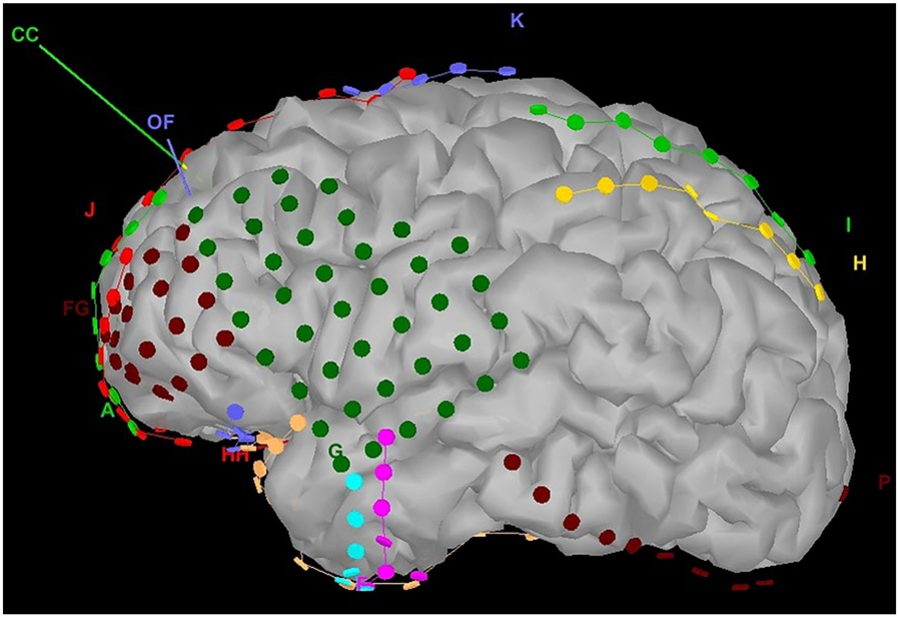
Reconstruction of electrodes on a mesh of a preimplantation 3-D reconstruction of the patient's own MRI (case 7)
